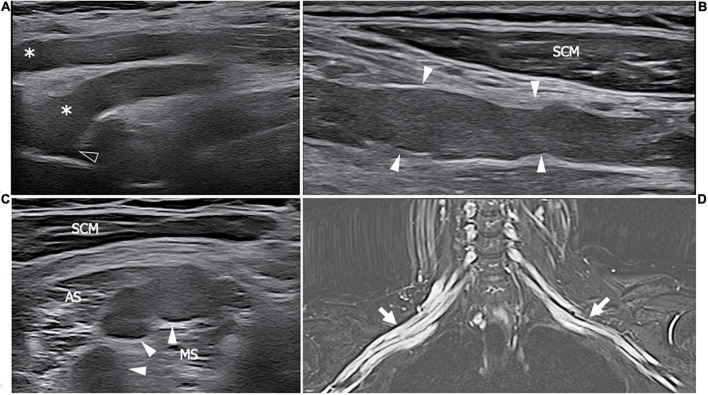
This figure shows three 18 MHz HRUS images (A–C) and a T2-weighted with fat-suppression magnetic resonance image (D) of a 50 years old female affected by SS who developed a predominant-upper limb sensory-motor demyelinating neuropathy. (A) A long-axis view of brachial plexus roots (white asterisk) at the exit from the intervertebral foramens (empty arrowhead) is obtained: the massive hypoechoic enlargement of the nerves with the complete loss of the typical fascicular echotexture is observed; these findings extend also more distally at the level brachial plexus trunks and divisions as seen in (B). Also in the latter, the long axis view depicts the irregular profile of the nerves (white arrowheads), with areas of thinning and area of nerve enlargement, findings which are typical of a CIDP neuropathy. (C) A short axis view of the pathological nerve trunks (white arrows) in the interscalenic space is shown. (D) The MR coronal view of the neck and upper thorax demonstrates a massive, irregular enlargement of the brachial plexus bilaterally (white arrows); the increased T2-signal of the brachial plexus roots, trunks, divisions and cords is due to an intraneural edema consequential to the nerve inflammation. SCM, sternocleidomastoid muscle; AS, anterior scalene muscle; MS, middle scalene muscle.
